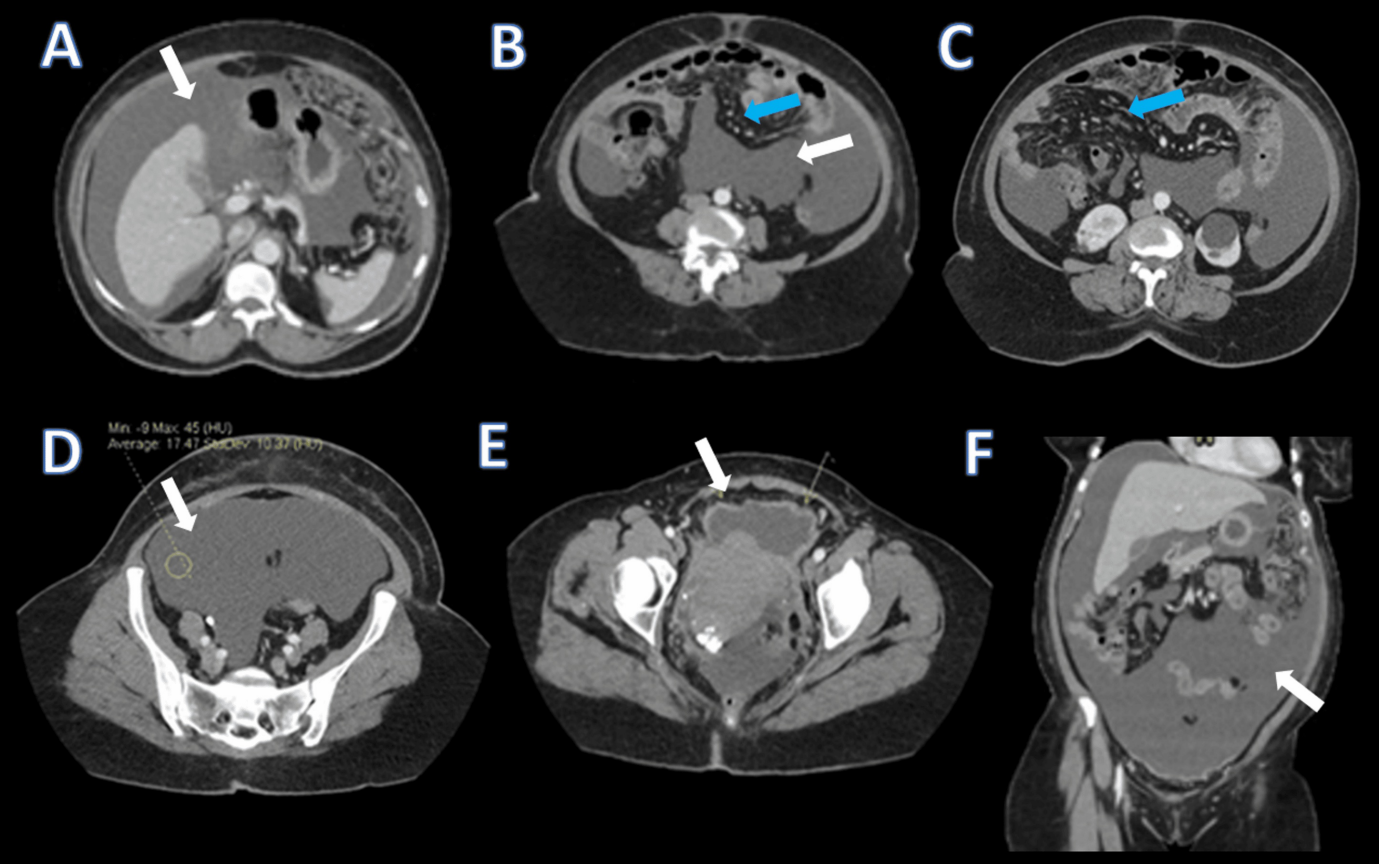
Contrast-enhanced CT of the abdomen and pelvis demonstrating diffuse peritoneal disease (A–C) Axial contrast-enhanced CT images of the upper and mid abdomen showing moderate-to-large volume ascites (white arrows) with diffuse omental and mesenteric reticulation consistent with omental caking (blue arrows). (D–E) Axial pelvic CT images demonstrating large-volume ascites and peritoneal thickening along the anterior pelvic peritoneum (white arrows), without evidence of an adnexal mass. (F) Coronal reconstruction demonstrating extensive intraperitoneal fluid distribution (white arrow) and diffuse peritoneal involvement.
